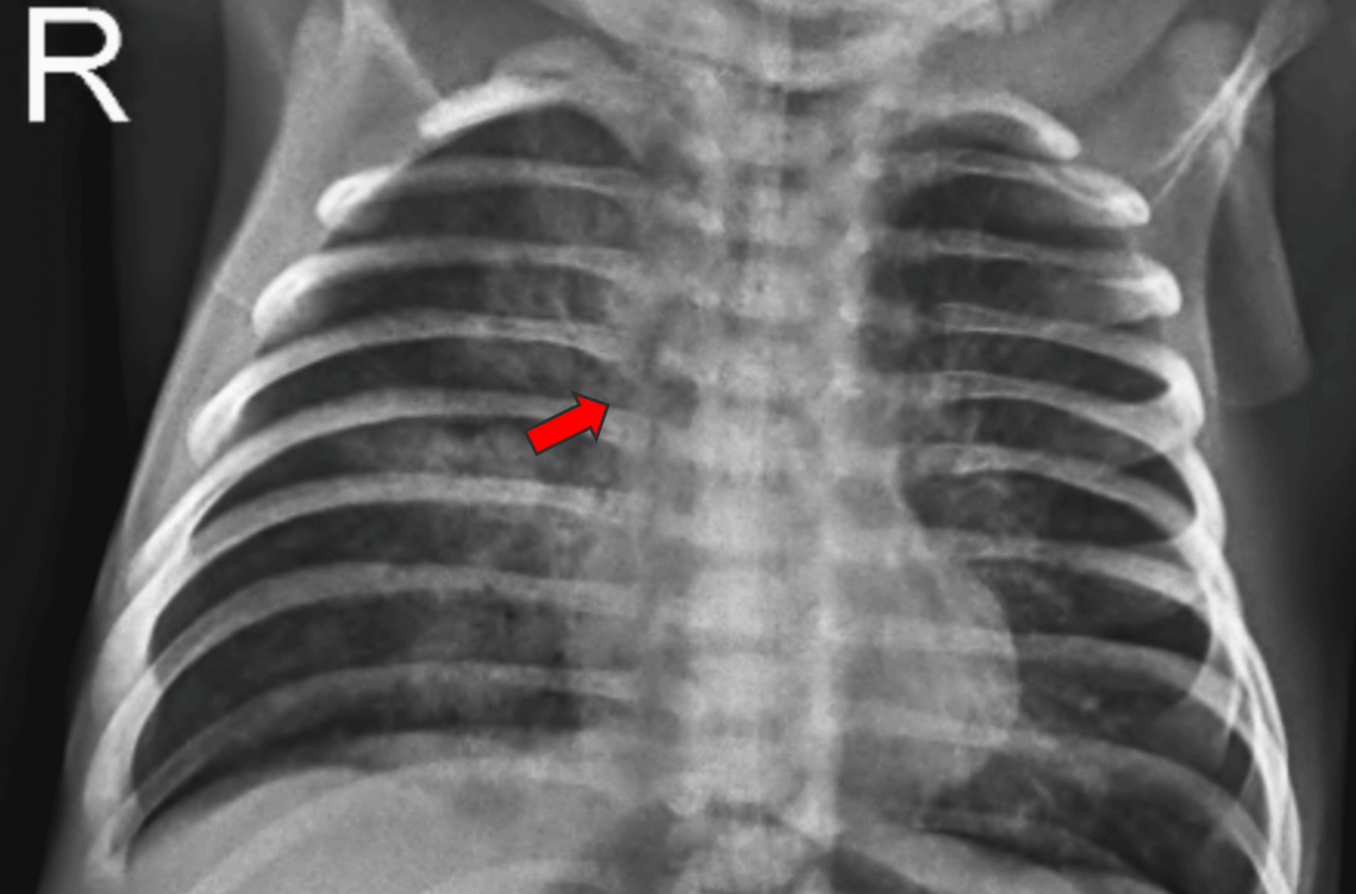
Chest X-ray taken 10 minutes after giving contrast showing the clearance of contrast (red arrow)
